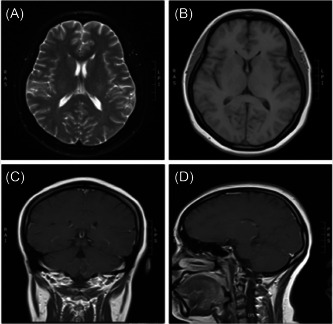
Cranial magnetic resonance imaging (MRI) examination. T2 FLAIR imaging (A), T1 imaging (B), T1 coronal (C), and T1 sagittal (D) showed no abnormal signals in the hippocampus and brain.
